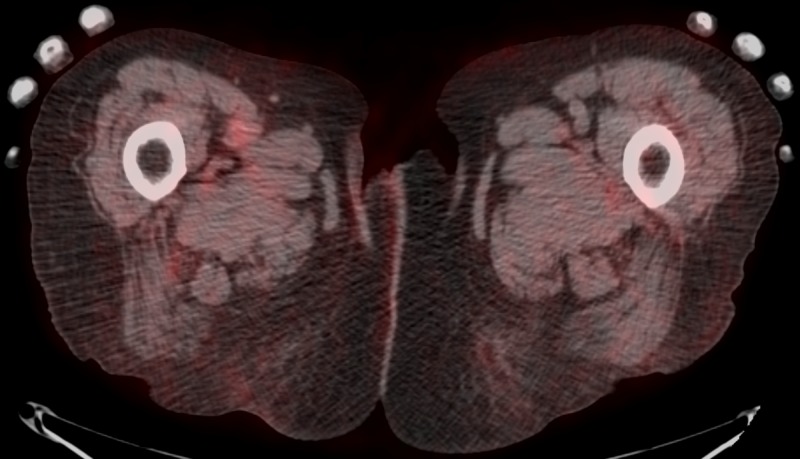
PET/CT December 28, 2015 positron emission tomography-computed tomography: PET/CT
